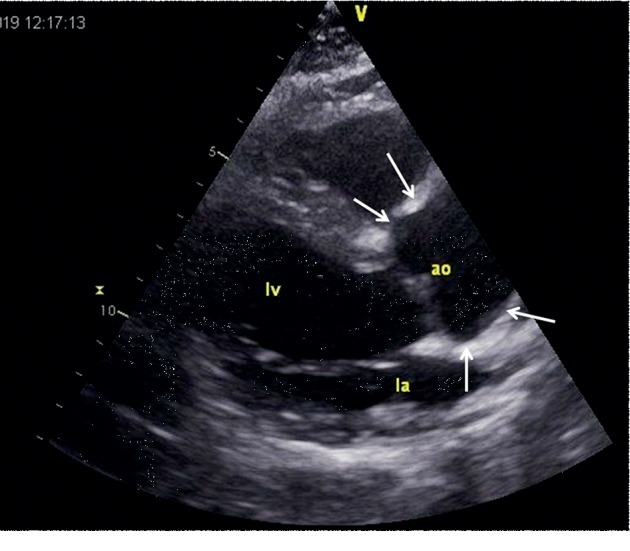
Echocardiographic parasternal long axis view showing the ectatic ascending aorta, measuring 37 mm in diameter.
